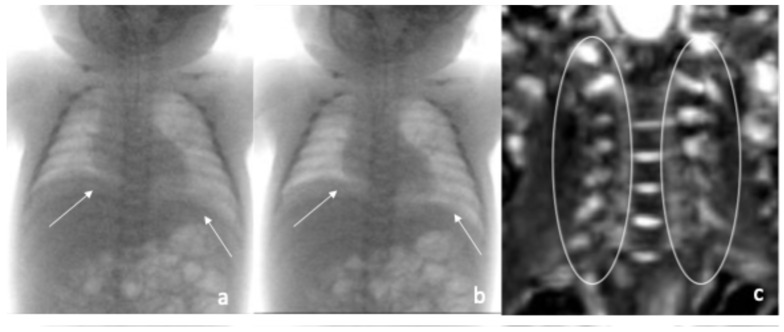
(a): Fluoroscopic evaluation of diaphragm in expiratory phase: the white arrows show the poor diaphragmatic excursion in the two respiratory phases. (b): Fluoroscopic evaluation of diaphragm in inspiratory phase: the white arrows show the poor diaphragmatic excursion in the two respiratory phases. (c): Magnetic Resonance Imaging of the brachial plexus: the white circles show the roots of the brachial plexus with no sign of avulsion.
